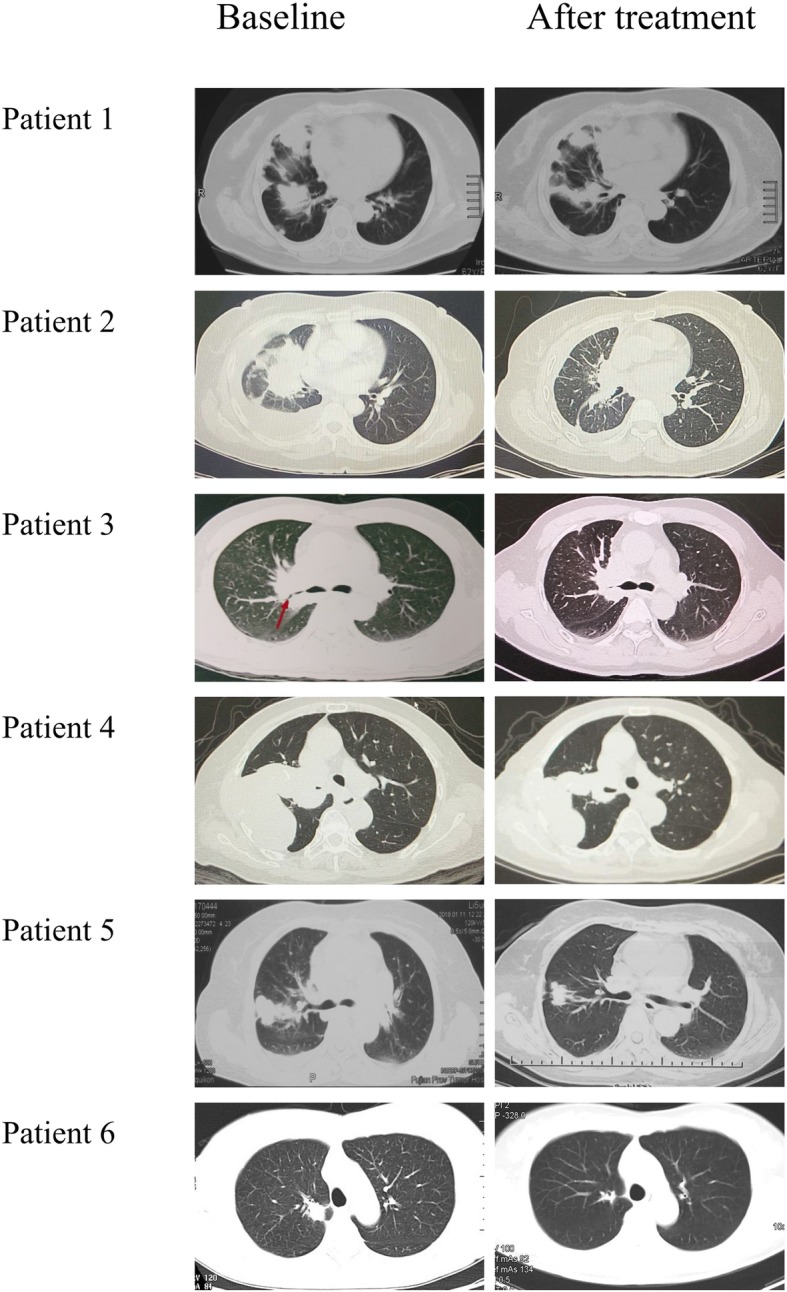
CT scans of the thorax performed before (baseline) and after osimertinib treatment (PR or SD). CT, computed tomography; PR, partial response; SD, stable disease
